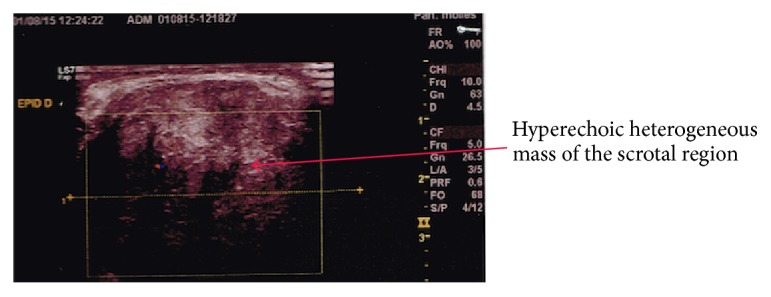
Ultrasonography showing a mass originating from the epidemic right chord.
